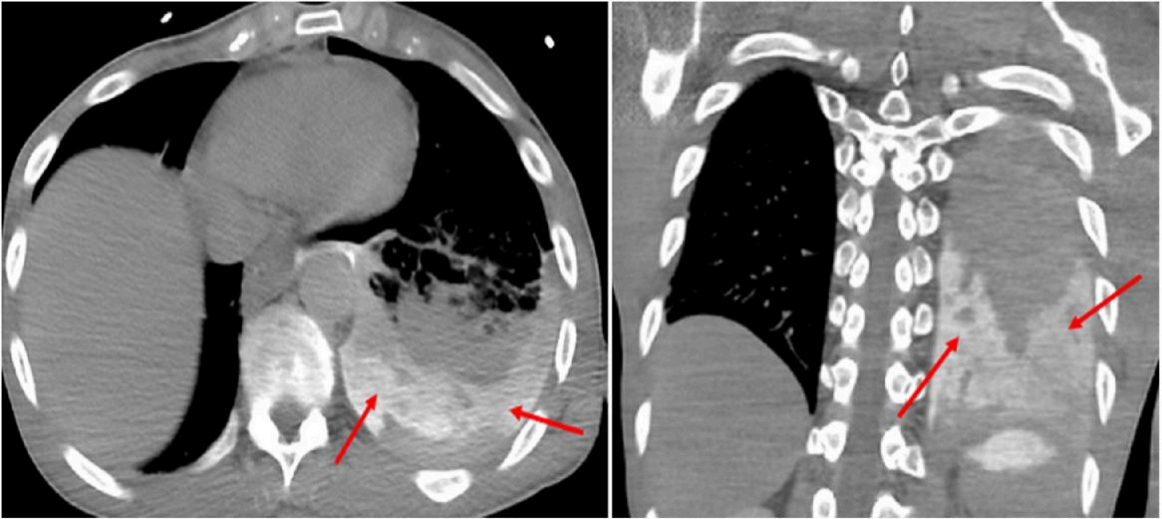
Chest computed tomography with oral contrast (axial and coronal computed tomography). Oral contrast within the left pleural space, consistent with oesophageal perforation (red arrows).
